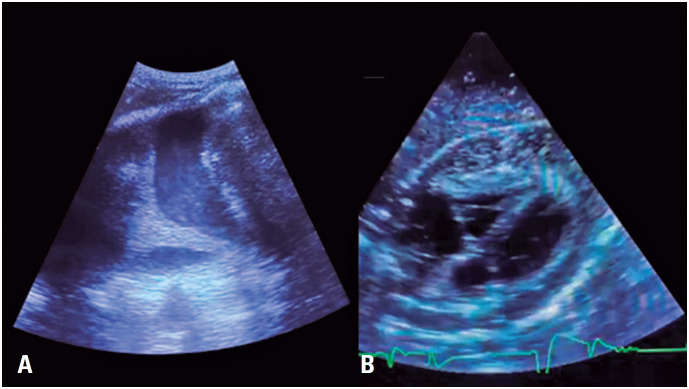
Point-of-care ultrasonography revealed right septated pleural effusion (A) and pericardial effusion with fine internal echoes (B)
